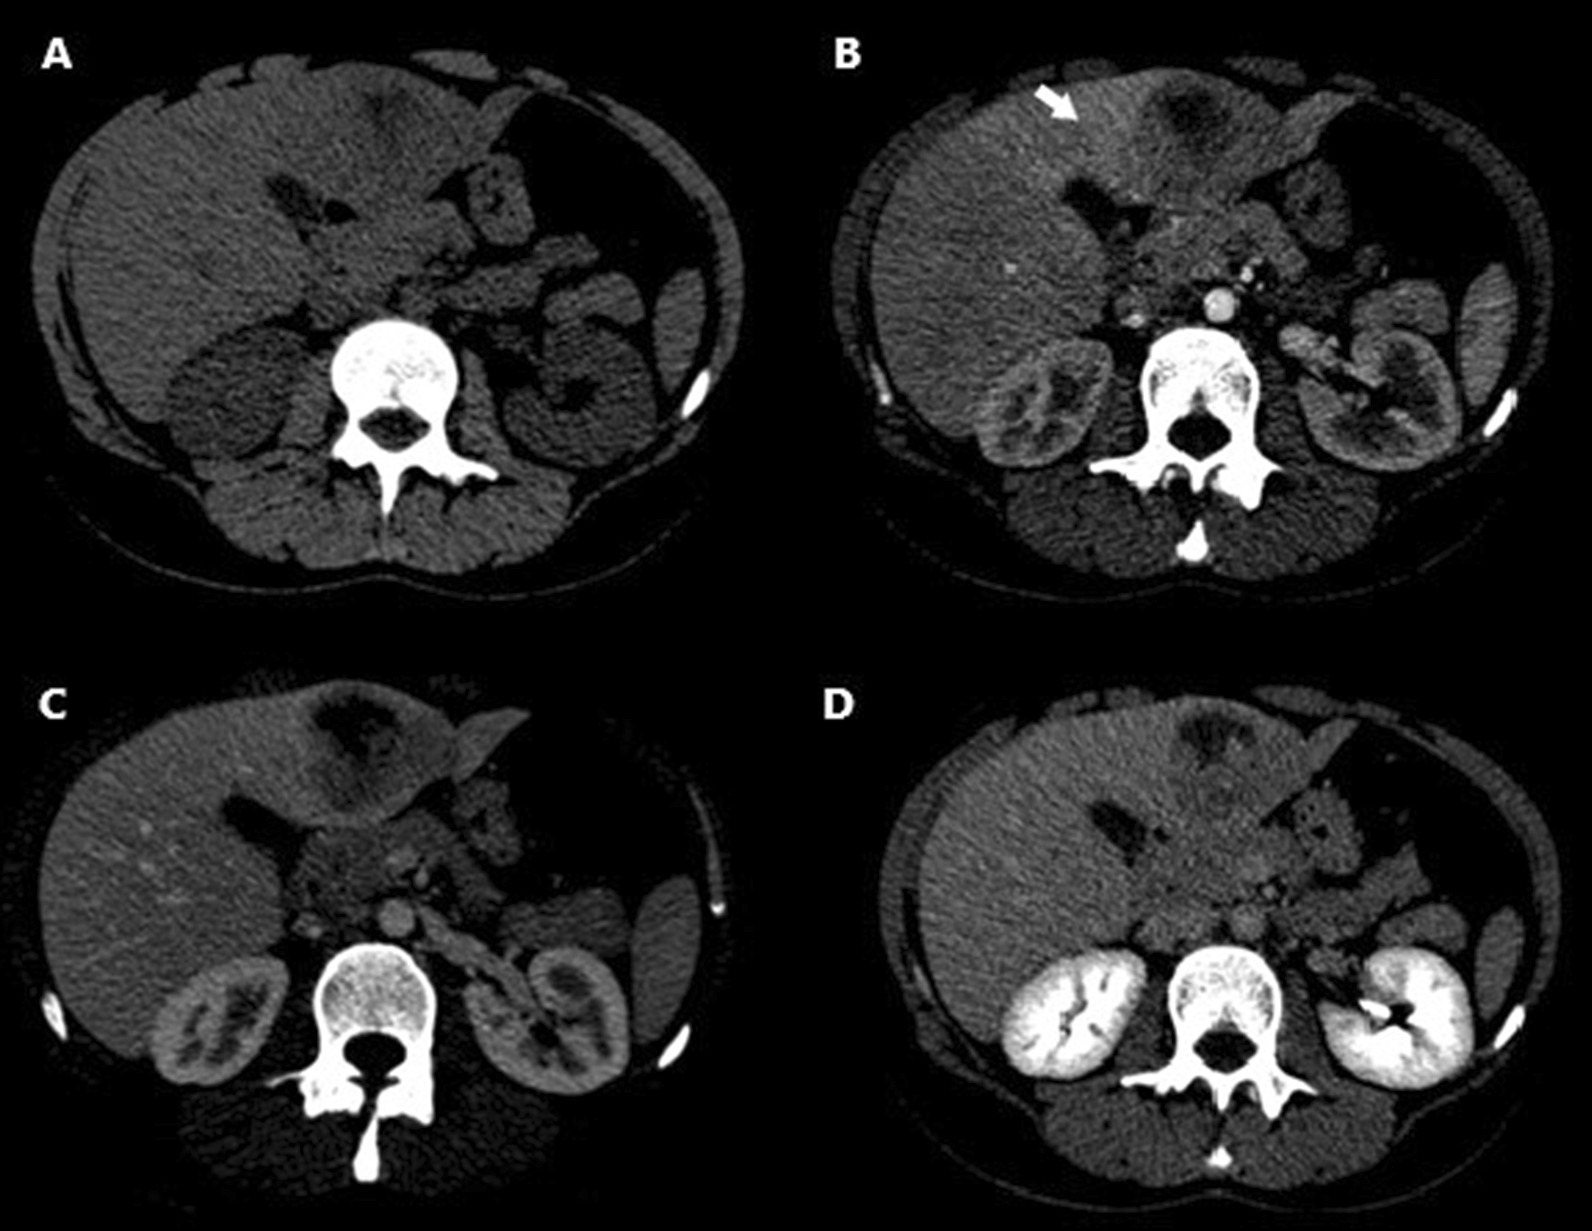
A Nonenhanced CT showing a heterogeneous hypodense mass, located in segment IVB of the liver. B–D Multiphase enhanced CT: arterial (B), venous (C), and delayed phases (D) revealing heterogeneous sustained hypoenhancement of the hepatic tumor. B Arterial phase demonstrating left lobe perfusion disorder (white arrow)
